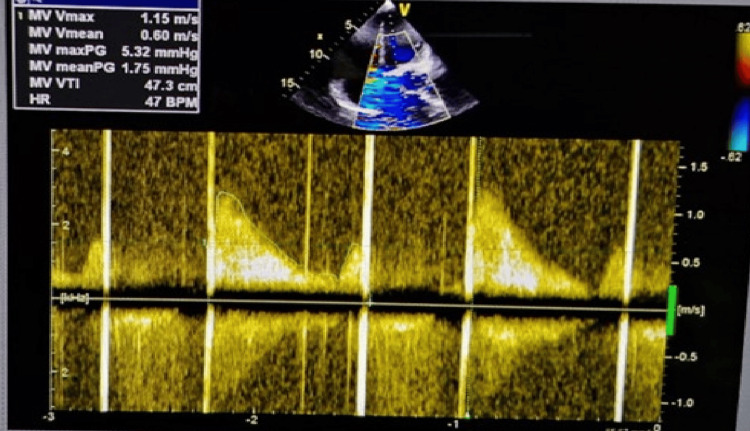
Post-thrombolysis Doppler ultrasound during first presentation Post-thrombolysis Doppler showing normalization of transmitral gradients (Pmax 5.32 mmHg, Pmean 1.75 mmHg), confirming non-obstructed mitral orifice. Pmax - peak gradient; Pmean - mean gradient
